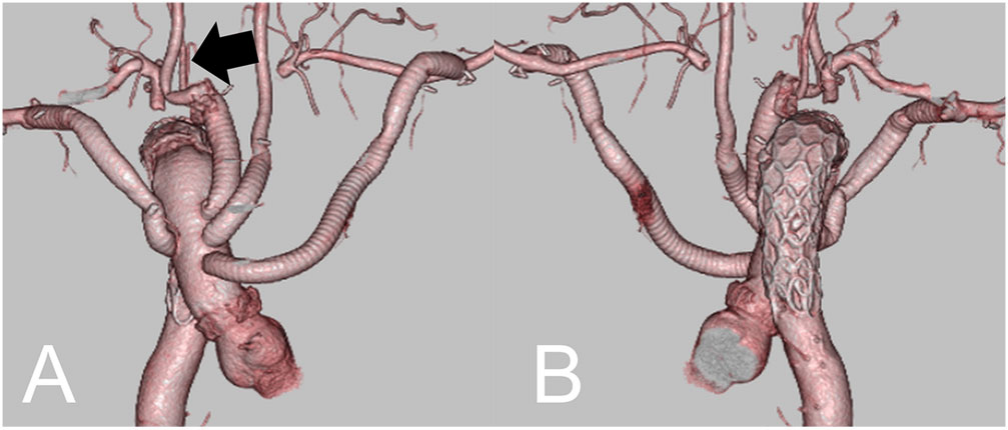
Postoperative three-dimensional computed tomography [(A) frontal view; (B) posterior view] shows good patency of the bypass graft and complete exclusion of the aneurysmal diverticulum. The black arrow points to the right vertebral artery.
